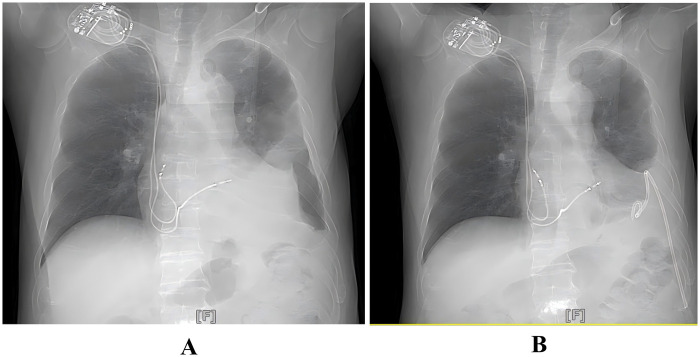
(A) chest CT at admission. (B) Chest CT after closed chest drainage.
